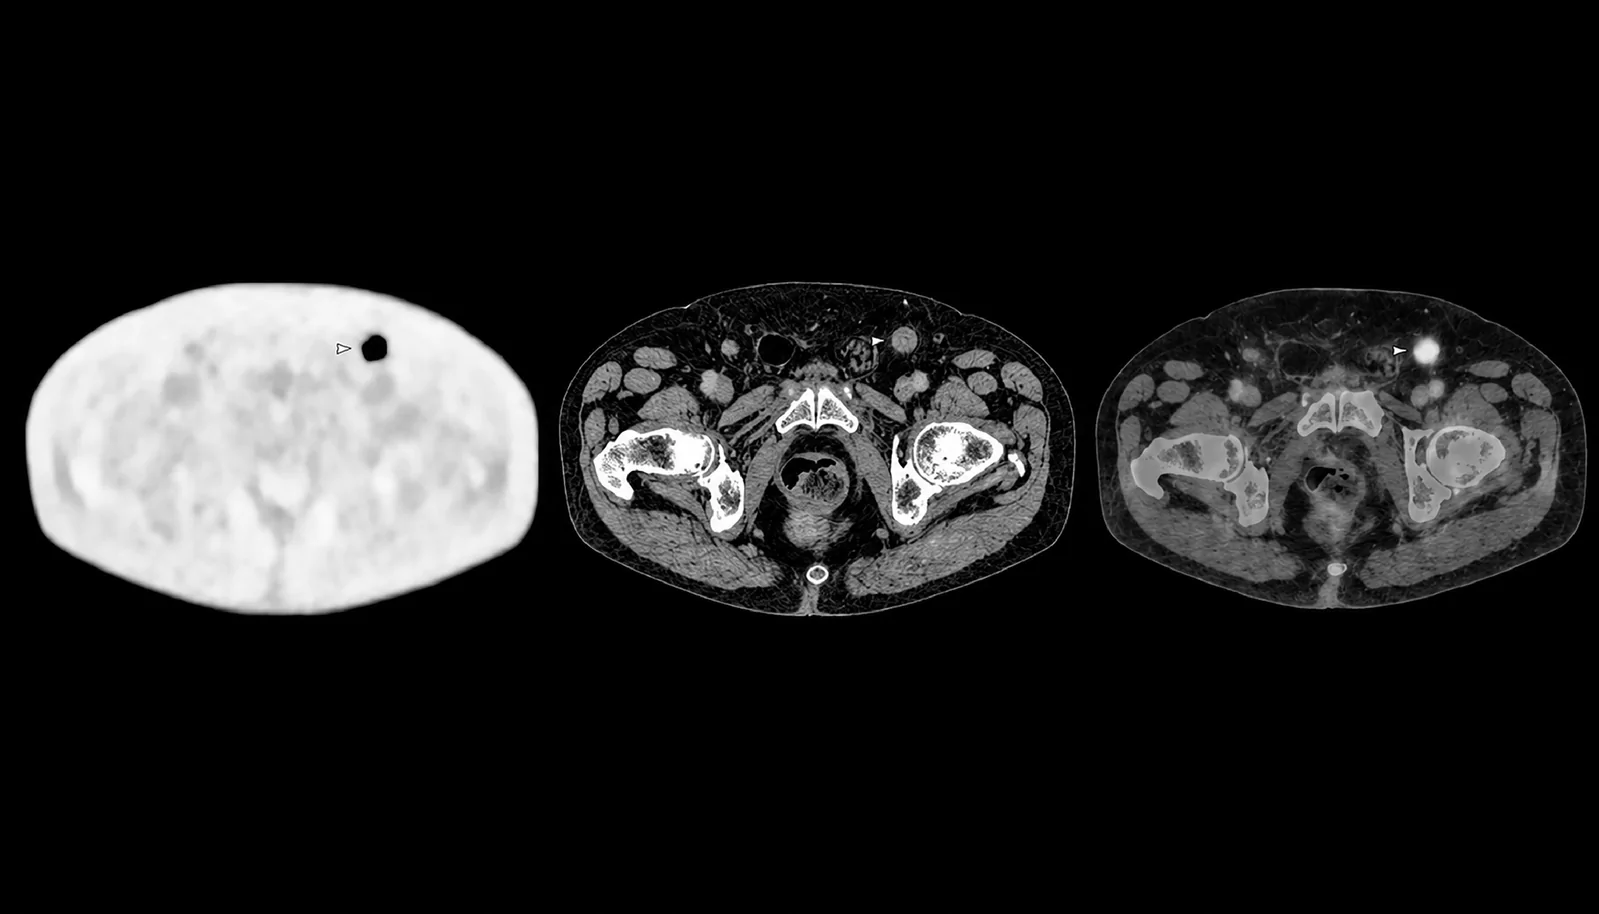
Axial PET, CT and Fused PET/CT images demonstrating FDG avid left inguinal lymphadenopathy (arrowheads).
